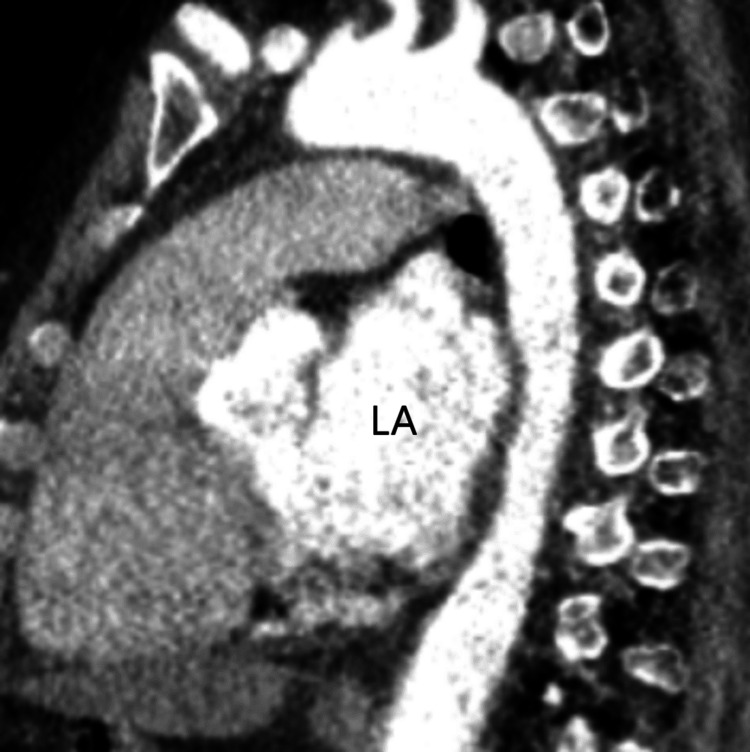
Sagittal computed tomography of the chest showing marked left atrium enlargement. LA: Left Atrium
